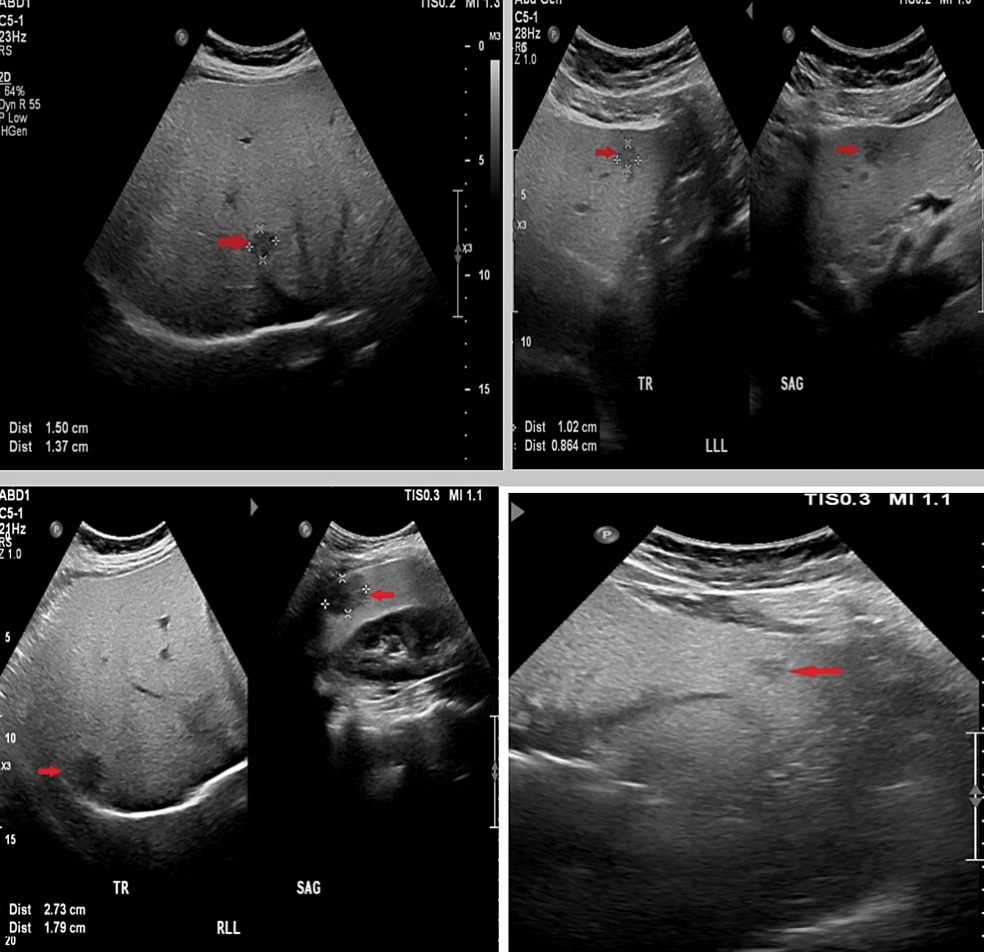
Multiple liver Ultrasound Images (A-D) reveal hyperechoic diffuse fatty liver with several focal hypoechoic masslike lesions (red arrows)
